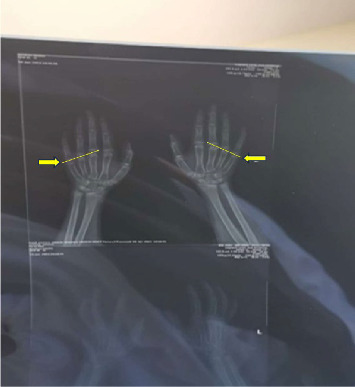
Hand radiograph showing slightly shortened 4th and 5th metacarpals as shown by yellow straight lines intersecting the 3rd metacarpal and dense sclerotic epiphyseal and metaphyseal bands, yellow arrows.
